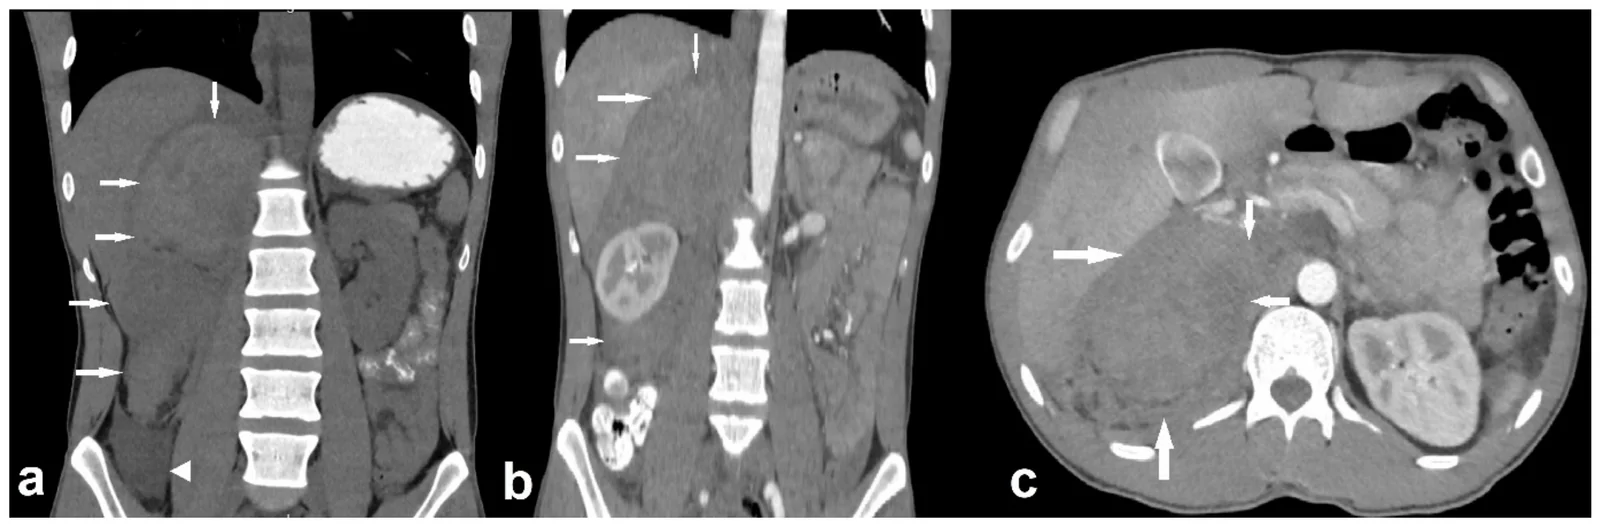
Initial CT images: (a) a coronal view without contrast showing a high-density hematoma (white arrows) in the right retroperitoneal space; (b) a coronal arterial phase image; and (c) an axial arterial phase image, all showing no signs of active bleeding (arrows). Additionally, free liquid is observed in the retroperitoneal space (arrowhead).
